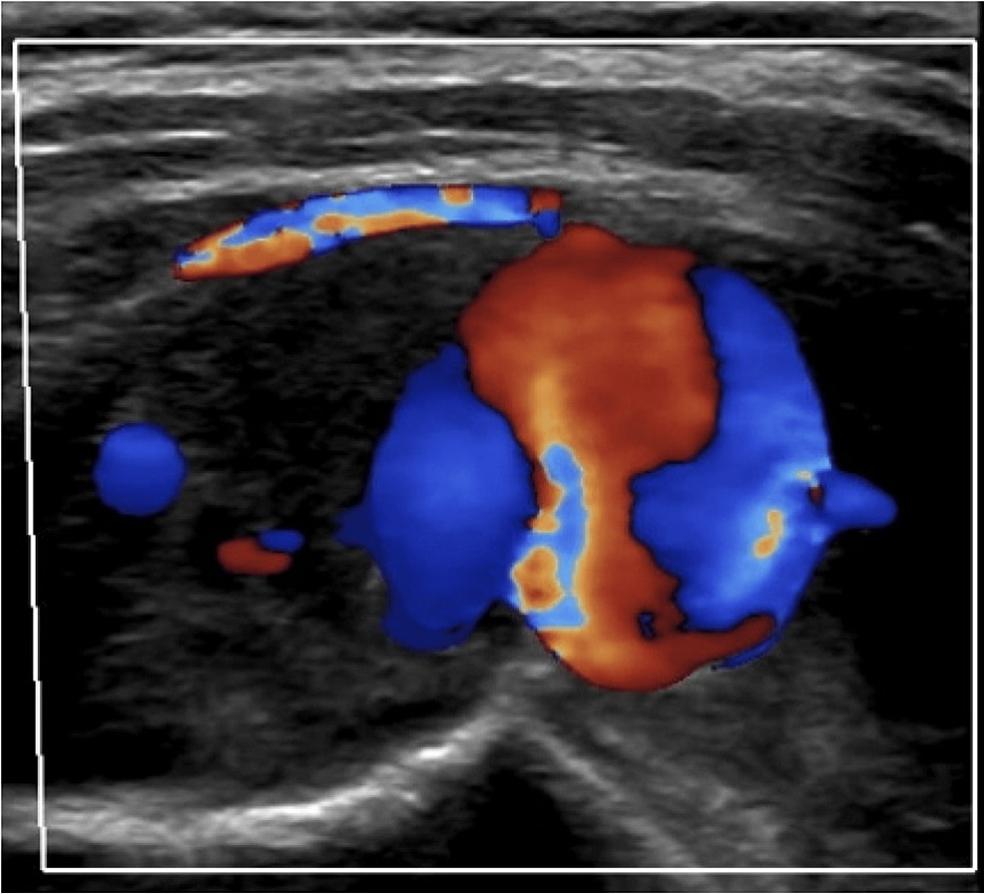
Ultrasonographic demonstration of heterogeneous hypoechoic mass with central vascular flow
